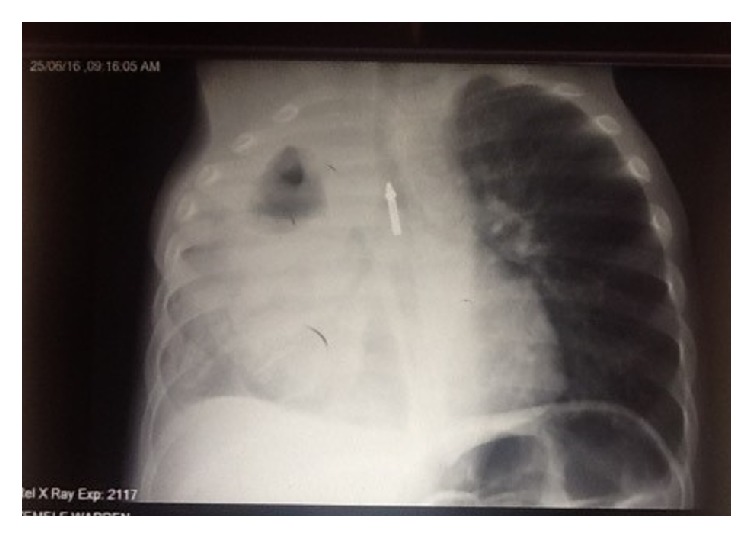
Chest X-ray of the boy at the time of the incident. The foreign body is visible in the chest. There is massive right pleural effusion and sign of esophageal perforation.
